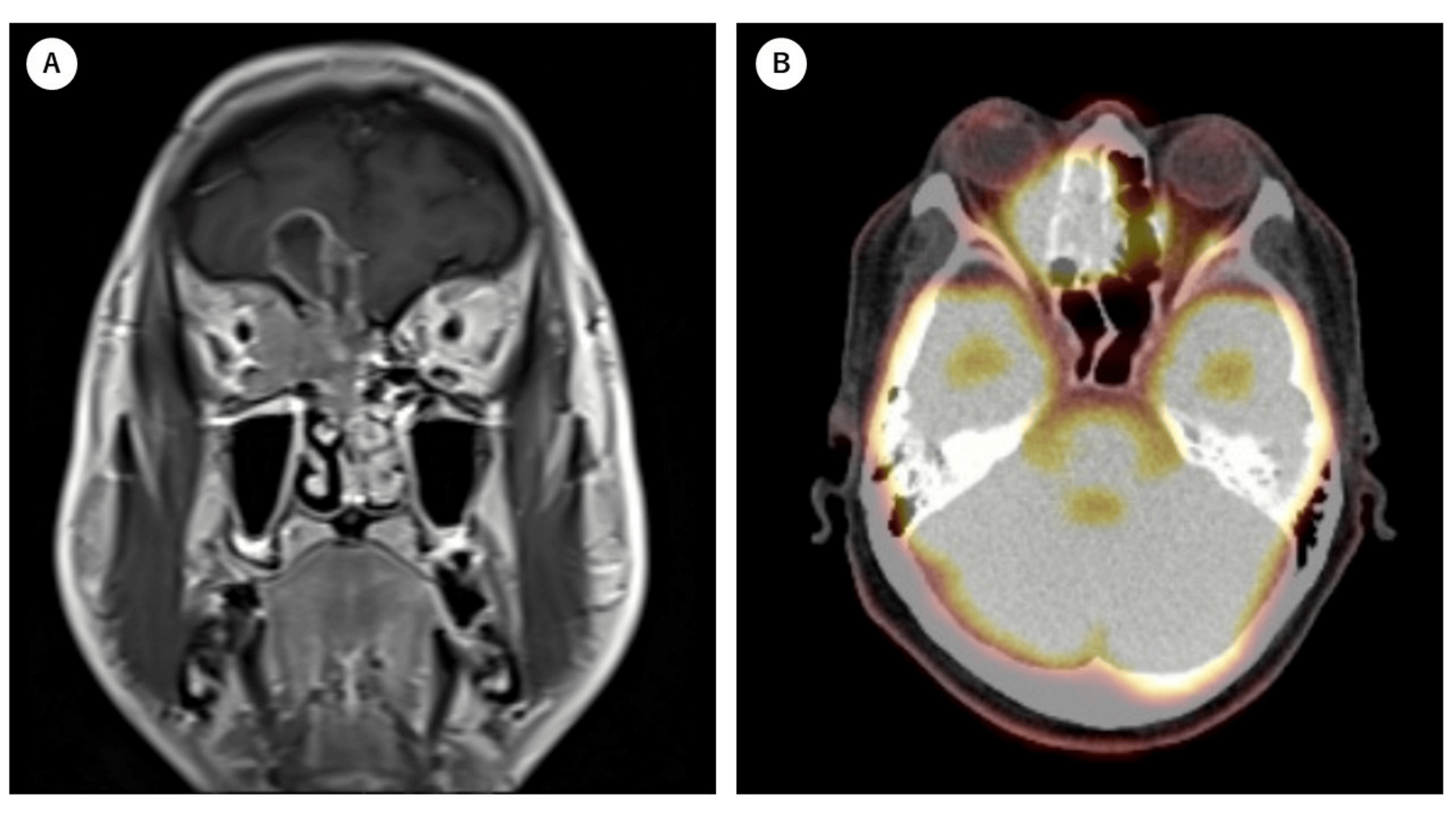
Pre-treatment MRI and FDG PET imaging A. MRI showing a T1-weighted tumor with gadolinium contrast enhancement in the right nasal cavity. The space-occupying lesion (SOL) with contrast enhancement at the edge of the right frontal lobe is shown. B. There is increased accumulation of FDG in the right paranasal cavity. FDG: fluorodeoxyglucose; PET: positron emission tomography
